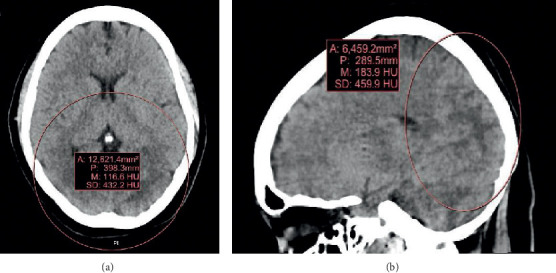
CT head without contrast showing increased low density in the left occipital white matter.
